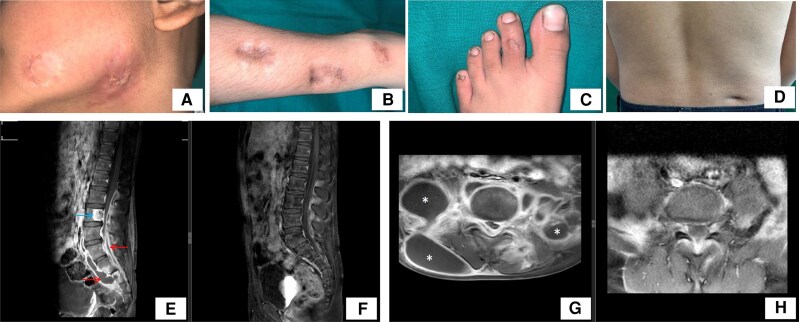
A–D*, Post-treatment photograph showing complete healing of all the lesions. *E* and *G*, Pretreatment MRI for comparison. *F*, Post-treatment MRI shows complete resolution of bony lesions except for a thin linear enhancement in the presacral region and ill-defined enhancement posterior to the spinal canal extending from L3 to S2 (sagittal plane). *H*, Post-treatment MRI shows a significant reduction in the abscesses with residual inflammation seen in bilateral paravertebral area and subtle inflammation around the epidural space (axial plane). Abbreviation: MRI, magnetic resonance imaging.
